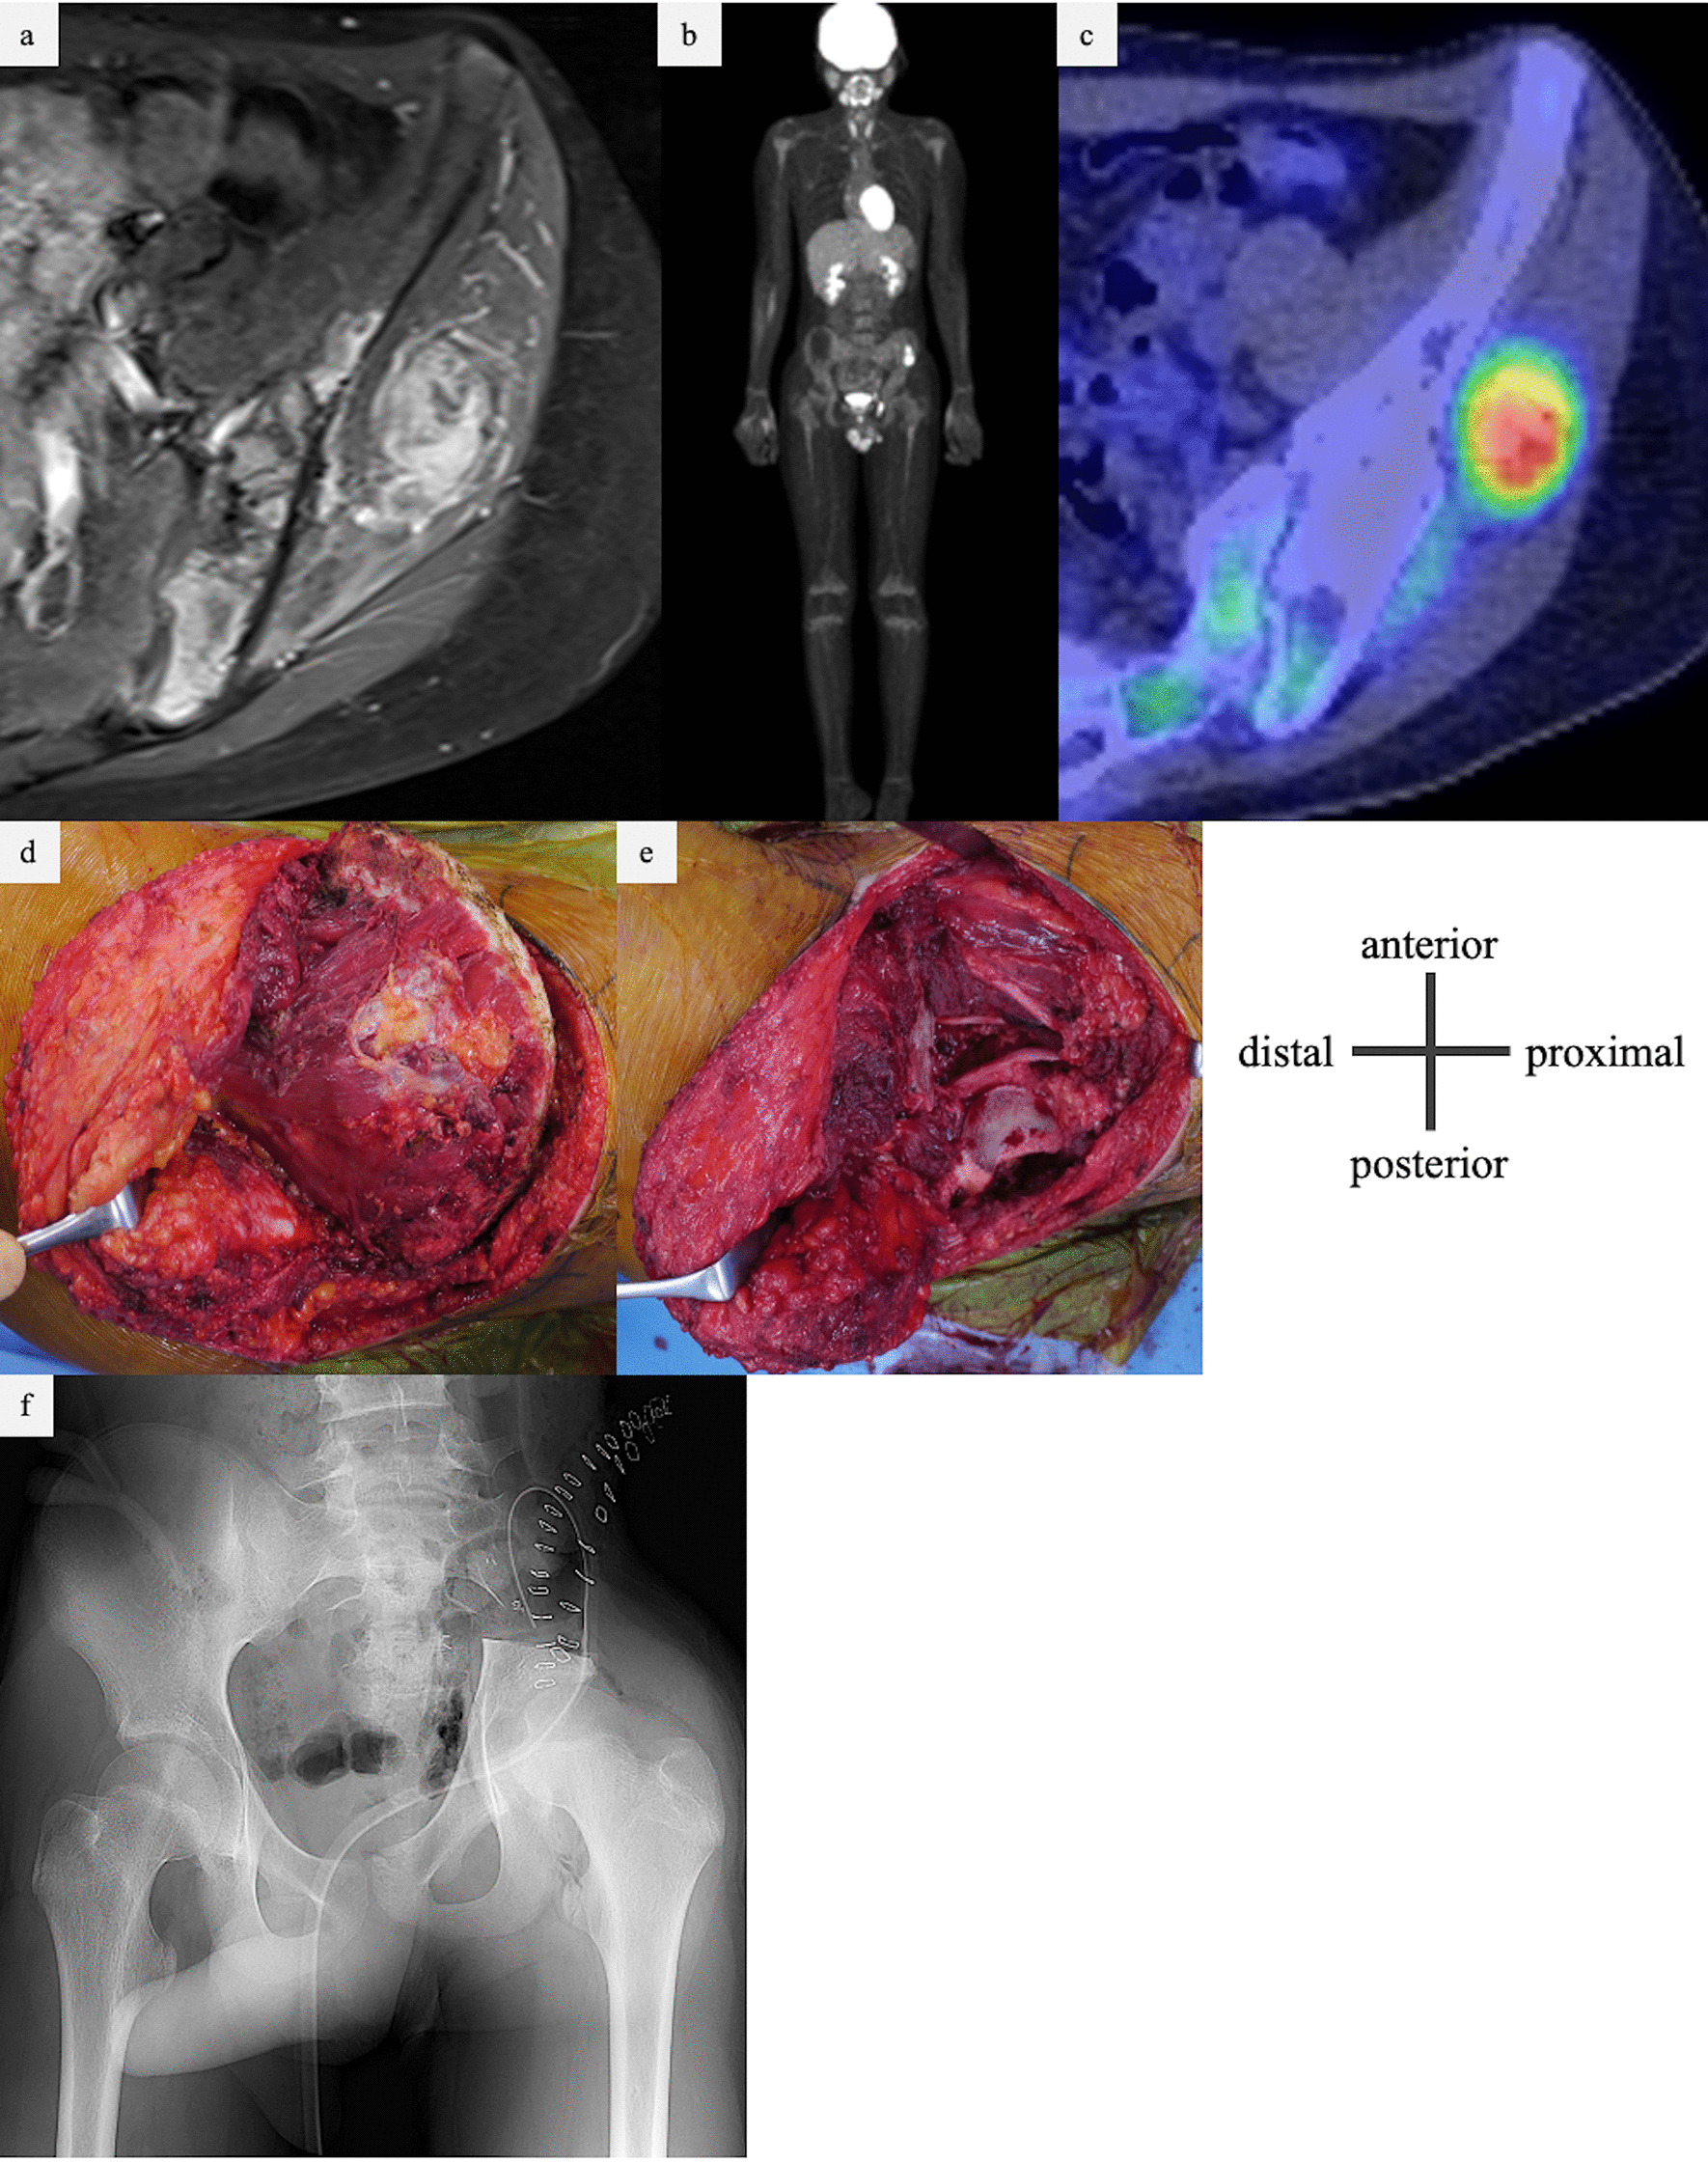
a–c Imaging findings after five courses of VDC/IE chemotherapy. a MRI showing the presence of an extra-bone mass within the gluteus medius muscle, although reduced in size. b, c PET–CT showing residual accumulation of primary tumor but disappearing of metastases. d, e Intraoperative findings of wide excision for the left iliac malignant bone tumor. d Before excision, an extra-bone mass was palpable within the gluteus medius muscle and resected together with the iliac wing. e Osteotomy was performed on the sacrum slightly medial to the sacroiliac joint and just above the acetabulum. f Postoperative X-ray. Pelvic ring reconstruction was not performed. PET–CT,  positron emission tomography–computed tomography; VDC/IE, Vincristine doxorubicin cyclophosphamide/Ifosfamide etoposide
